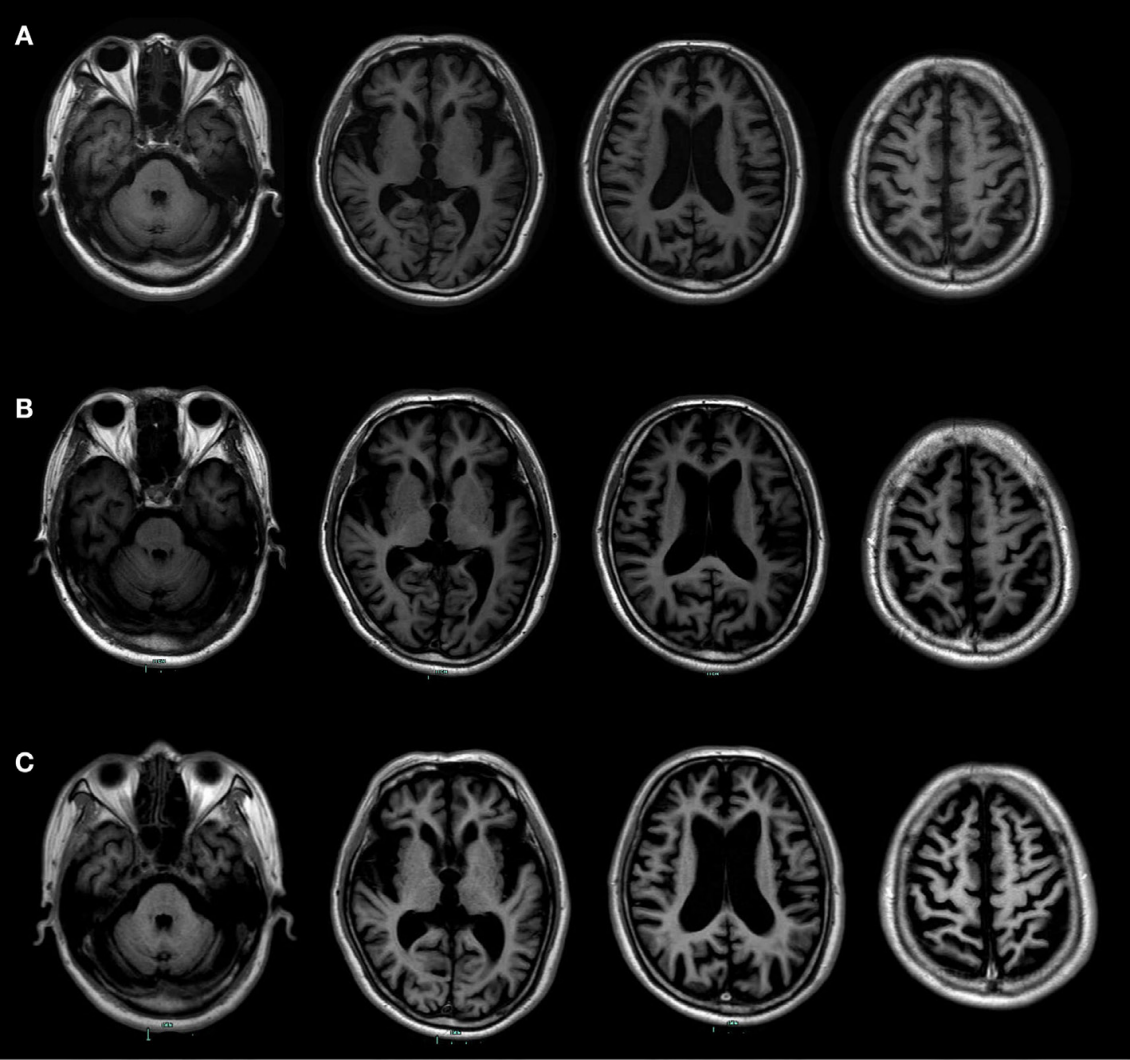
Longitudinal structural MRI scans showed progressive brain atrophy. (A) It was performed at the age of 73. (B) It was performed at the age of 74. (C) It was performed at the age of 76. Those follow-up MRI scans indicated asymmetric atrophy of the frontal, temporal, and parietal lobes that was more pronounced on the left side of the brain, especially on the left inferior frontal and insular.
